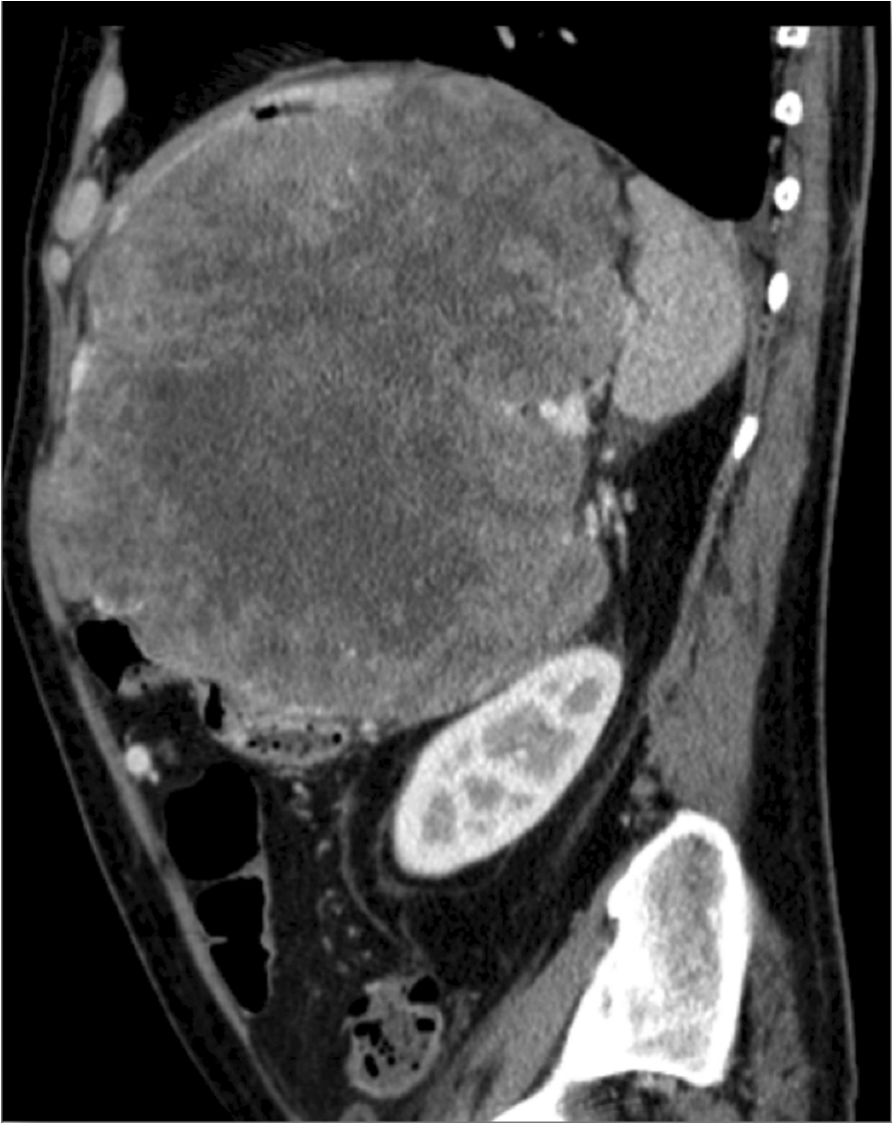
Sagittal computed tomogram, showing the extent of tumor and the compression of the left colonic flexure.
